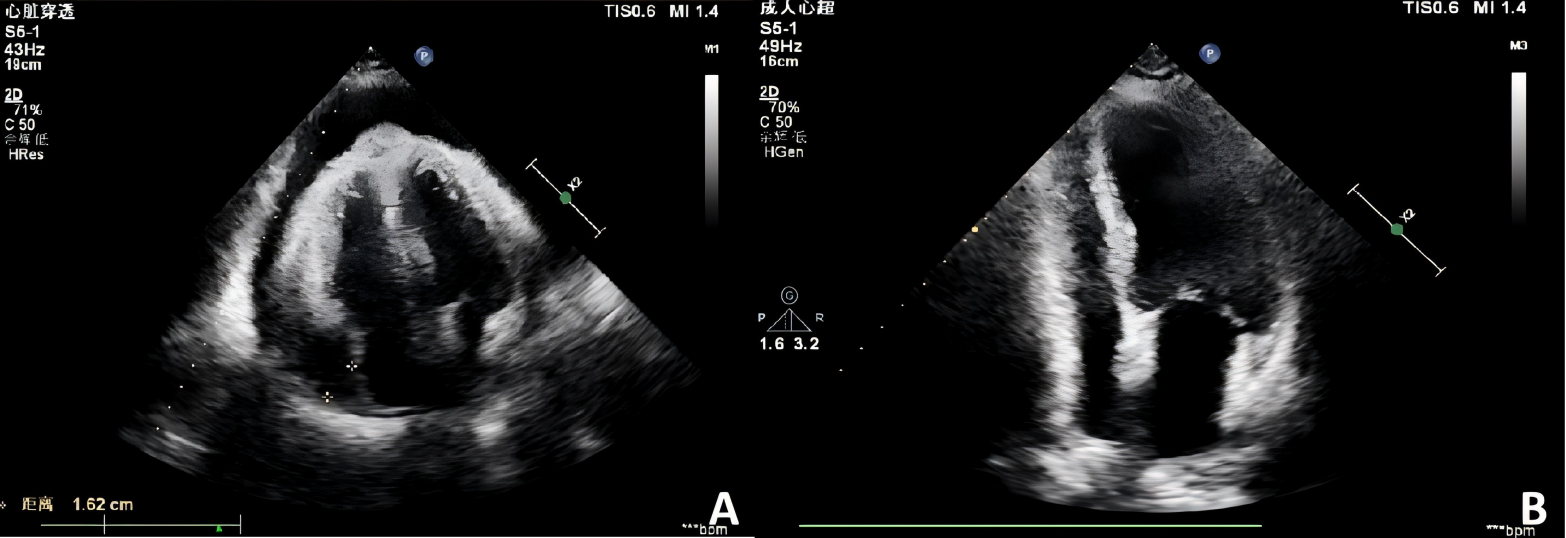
Echocardiographic assessment of pericardial effusion. (A) Initial echocardiogram (October 2022) reveals a moderate to severe pericardial effusion. (B) Follow-up study (June 2024) demonstrates complete resolution of the effusion.
